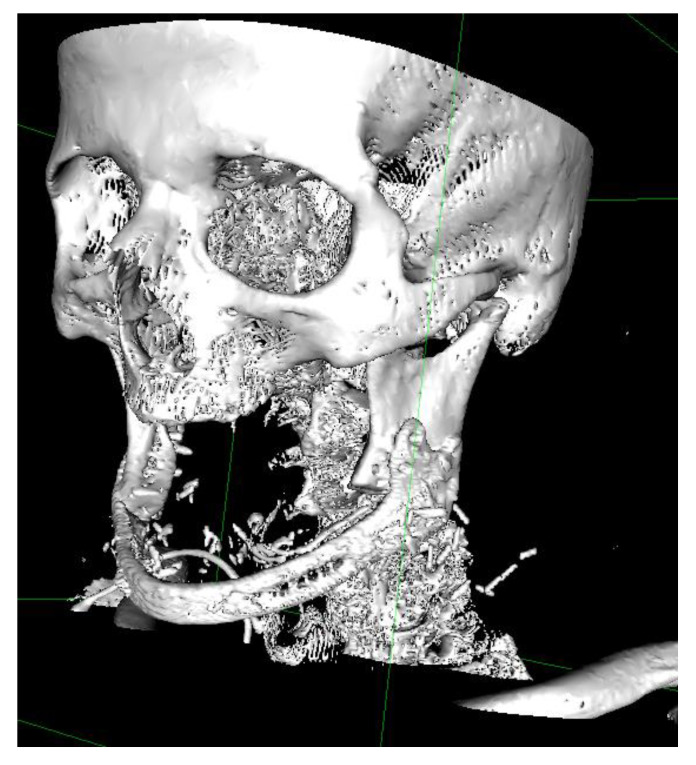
Postoperative CT-scan 3D reconstruction in a patient treated for symphysis BRONJ.
